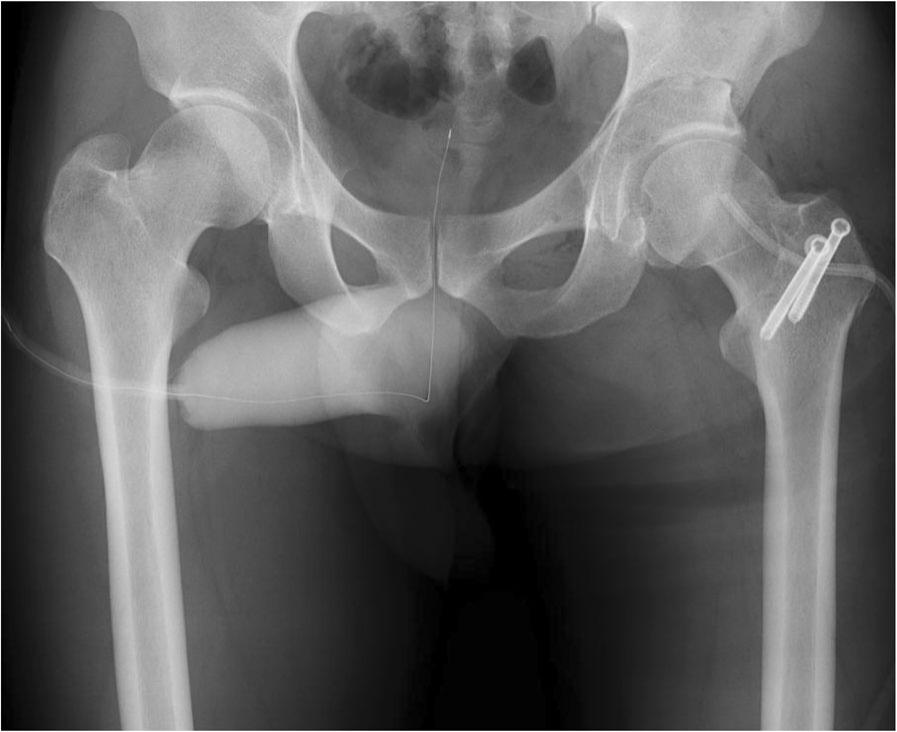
Intraoperative anteroposterior radiograph confirms the disappearance of the crossover sign and the presence of adequate posterior bone support
